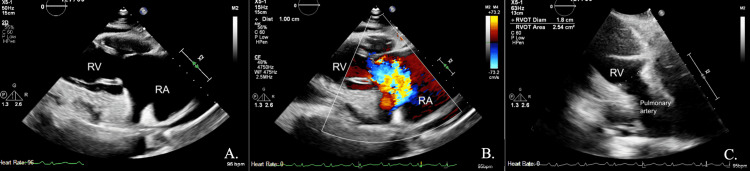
Transthoracic echocardiogram of the tricuspid valve RA, right atrium; RV, right ventricle (A) Right ventricular inflow view demonstrating retracted anterior and posterior leaflets of the tricuspid valve, resulting in non-coaptation at the end-systole. (B) Color flow Doppler image showing “wide-open” tricuspid regurgitation as a result of non-coaptation. (C) Right ventricular outflow tract showing a decreased diameter (1.8 cm) across the pulmonic valve, resulting in pulmonic stenosis (normal: 2.1-3.1 cm)
